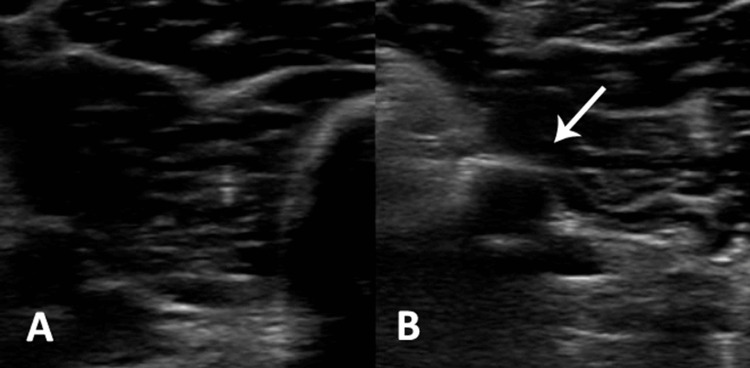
Ultrasound of the left pelvic limb In image (A), we can observe a completely dilated deep femoral vein. In image (B), on the other hand, we find a vein that does not collapse when pressure is applied (white arrow), which translates to the presence of a thrombus that is obstructing the vessel lumen.
